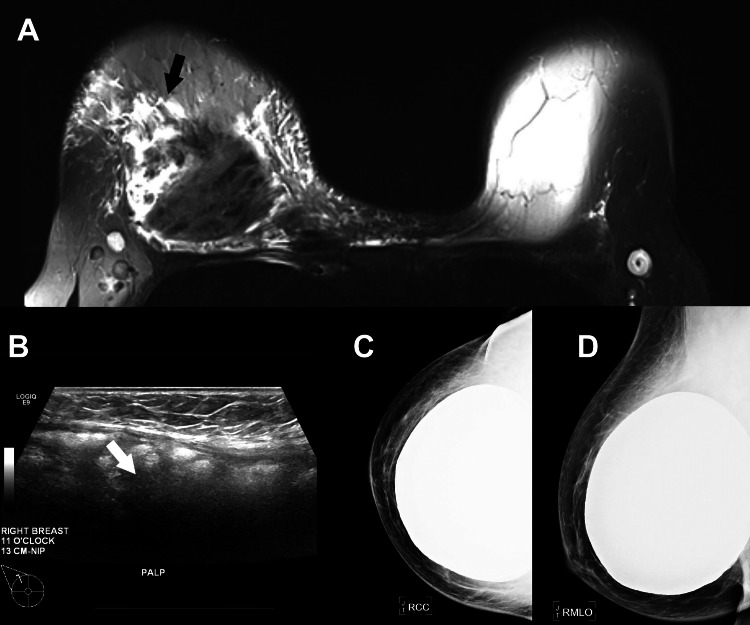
(A) Magnetic resonance imaging shows prepectoral silicone implant with intracapsular rupture, with free silicone and associated non-mass enhancement likely representing granulomatous reaction. (B) Ultrasound showing snowstorm appearance consistent with free silicone. (C) Craniocaudal and (D) mediolateral oblique mammogram views did not show free silicone (likely obscured by the overlying implant).
